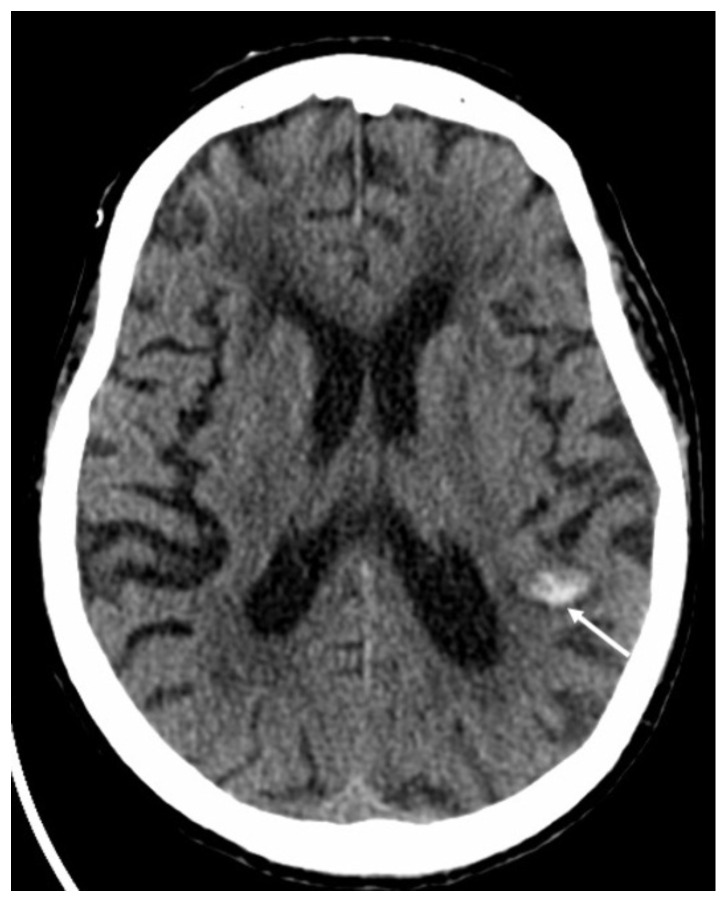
Axial computed tomography shows a small hemorrhage in the subcortical region of the left parietal region (arrow) in a patient with varicella zoster virus encephalitis.
